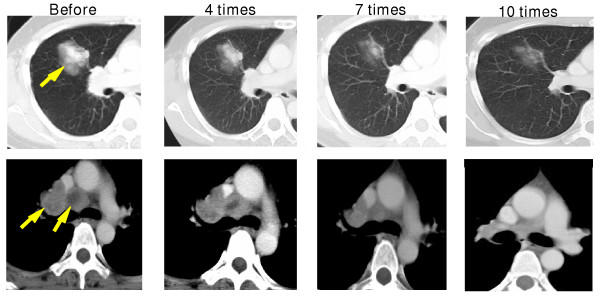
Impact of DC vaccines on metastatic lesions of the lung in responder patient 1. Upper and lower panels show a lung and hilar lymph node metastatic lesion (arrow), respectively. The CT scan was made before therapy and after 4, 7 and 10 DC vaccinations.
